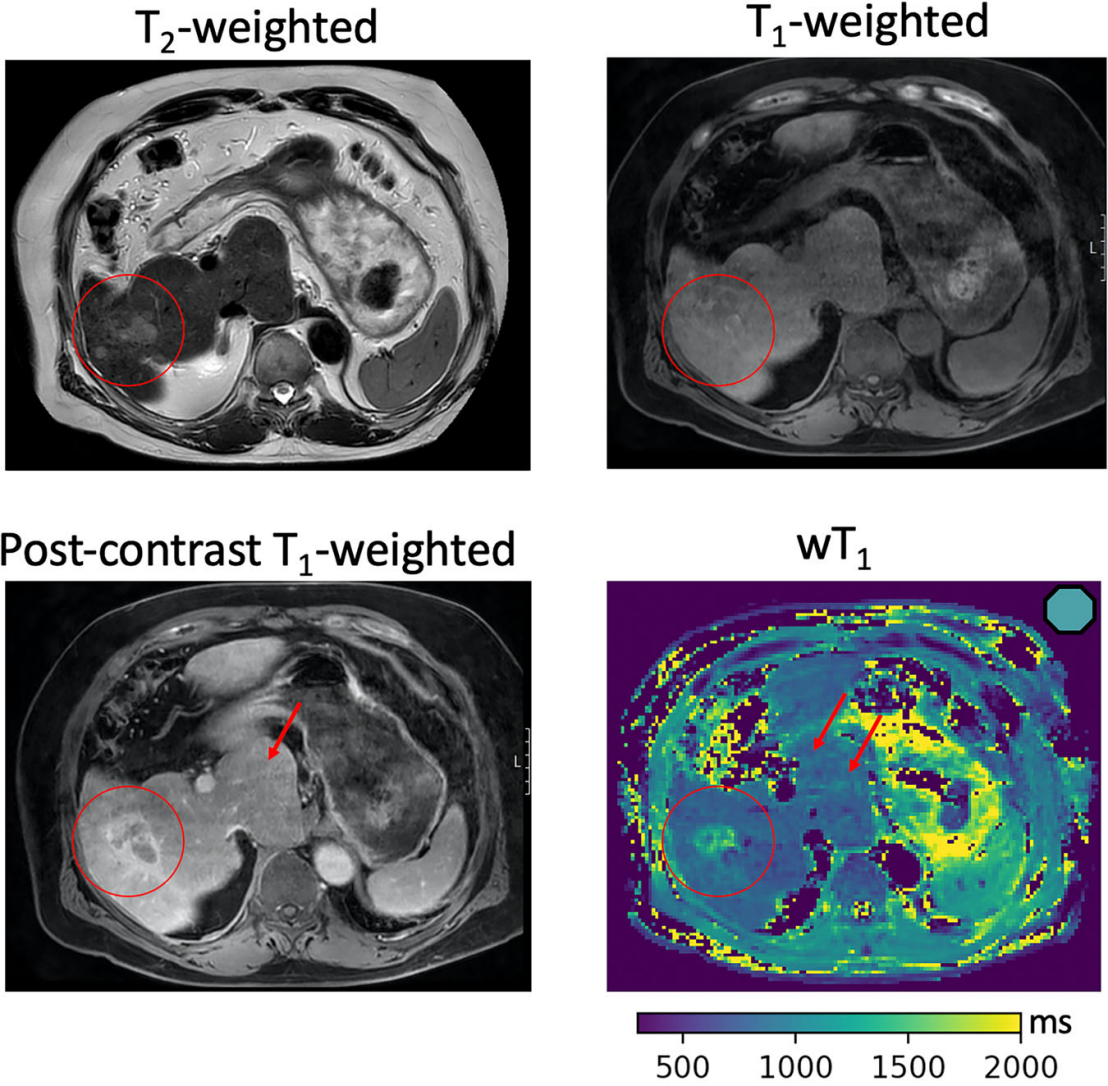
A 73-year-old male with unifocal HCC in S6 (3.0 × 1.8 cm). The HCC (circle) shows hyperintensity on the T2-weighted image, hypointensity on the T1-weighted image and enhancement and washout after gadolinium contrast in the post-contrast T1-weighted image. Reticulations in S1 (arrow) appear post-contrast, with early arterial phase enhancement. On the wT1 map, scars in S1 (arrows) show prolonged T1 relaxation, detectable without contrast agent. The wT1 and wT1_comp_ values of S1 (wT1_S1_ = 893 ms, wT1_comp-S1_ = 988 ms) are represented as a thick-edged octagon (right upper corner wT1 map) in Fig. 4c. HCC, Hepatocellular carcinoma; wT1, Water T1; wT1_comp_, T2*-compensated wT1
